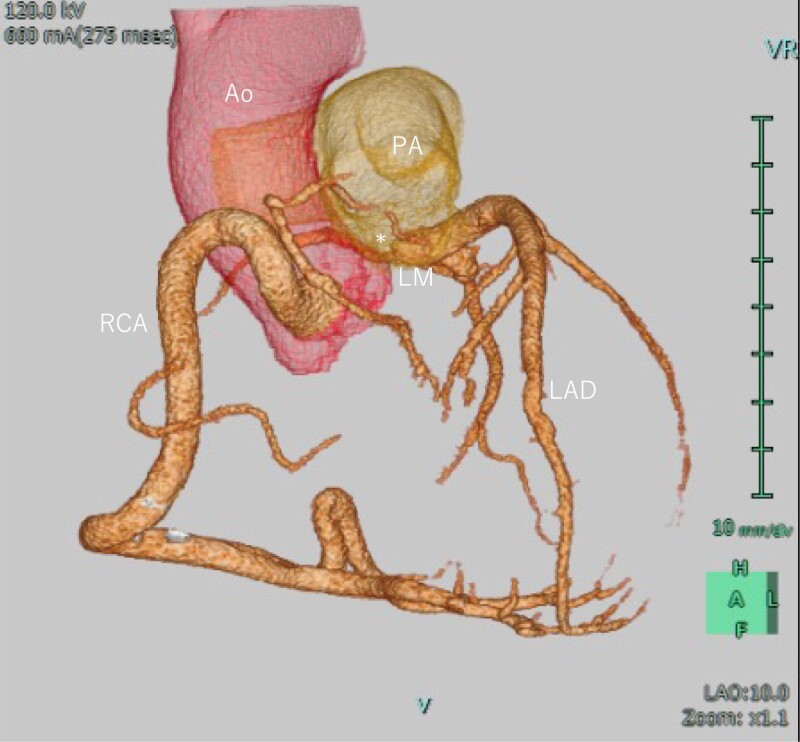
Pre-operative coronary computed tomographic angiography (CTA). The dilated RCA arising normally from the aorta and the left main coronary artery (LMCA) abnormally originating from the main pulmonary artery.
